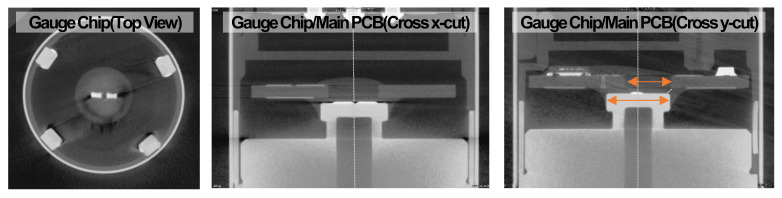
The structural analysis results of three-dimensional X-ray CT indicate that there are no structural abnormalities except for the intentional misalignment.
